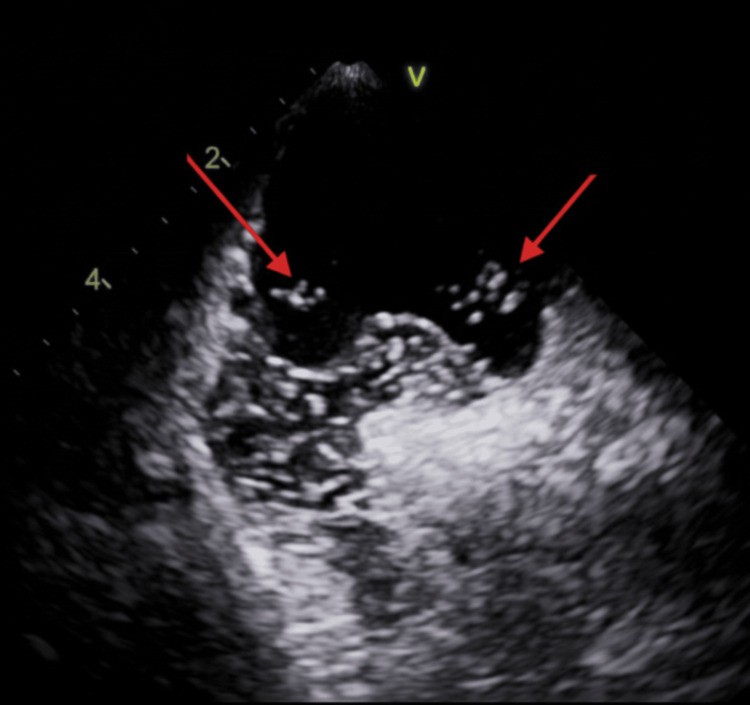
Bubble Study Demonstrating Right-to-Left Intracardiac Shunt Transesophageal echocardiographic bubble study demonstrating microbubbles entering the left atrium shortly after right atrial opacification, confirming right-to-left shunting through a patent foramen ovale, with red arrows indicating the microbubbles.
